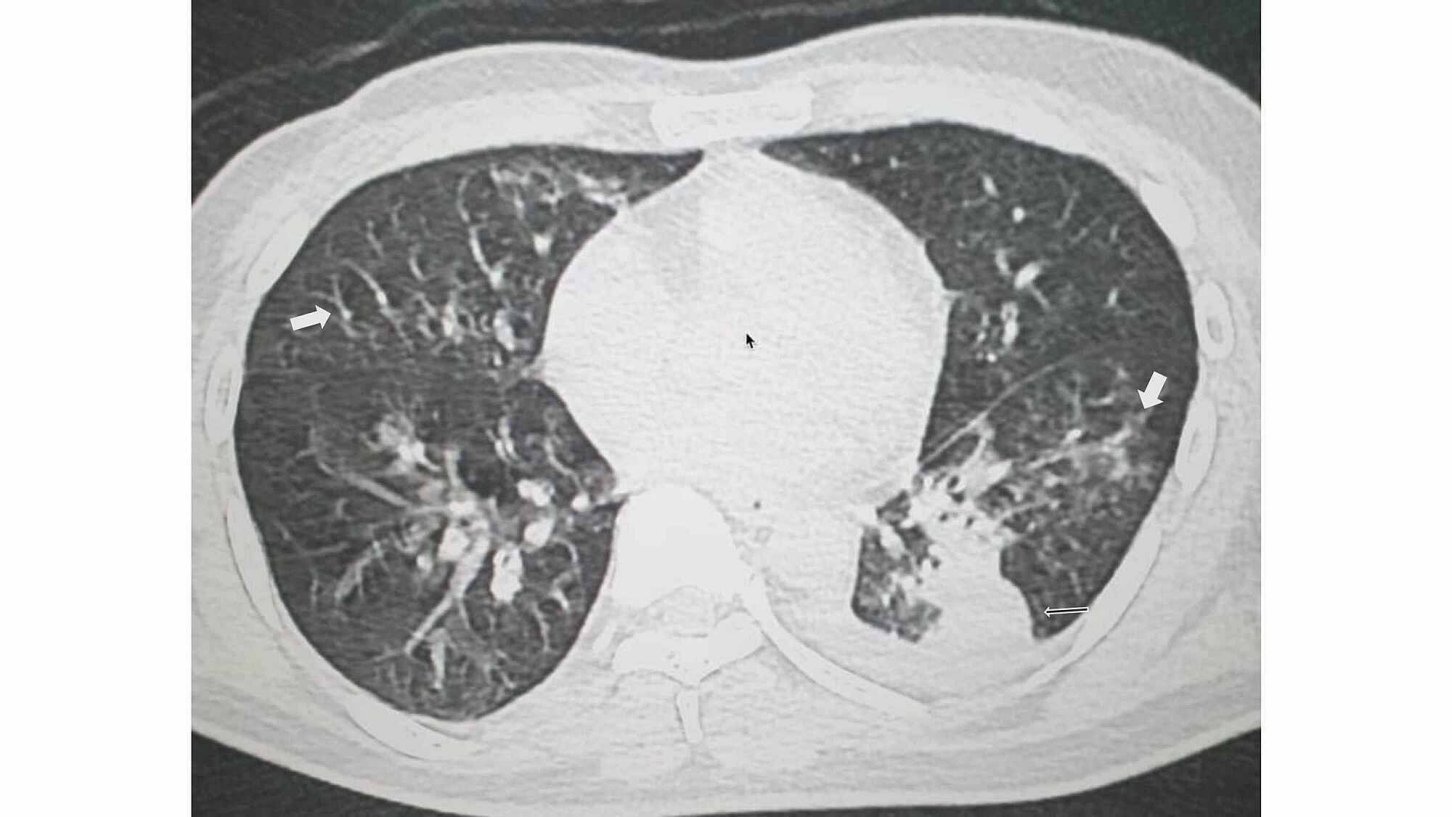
Non-contrast CT scan of the chest Bilateral ground-glass opacities with a tree-in-bud appearance are visible. A patch of consolidation in the left upper lobe and mild left pleural effusion can also be seen with the possibility of chemical or aspiration pneumonitis.
